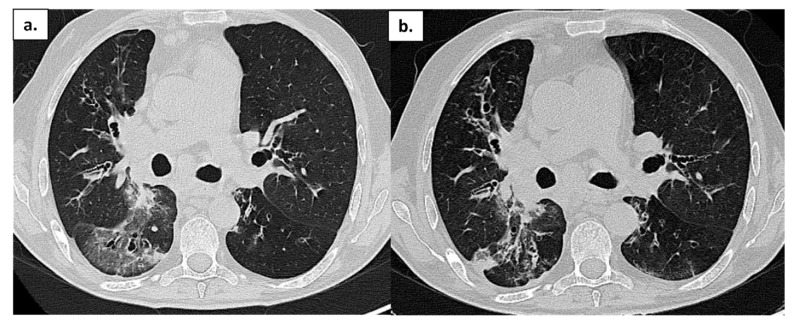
Patient 4: Chest CT in a patient with APDS-1 at onset of COVID-19 pneumonia (a) and after treatment (b); ground-glass opacities are reduced, but some areas of consolidation are still present.
